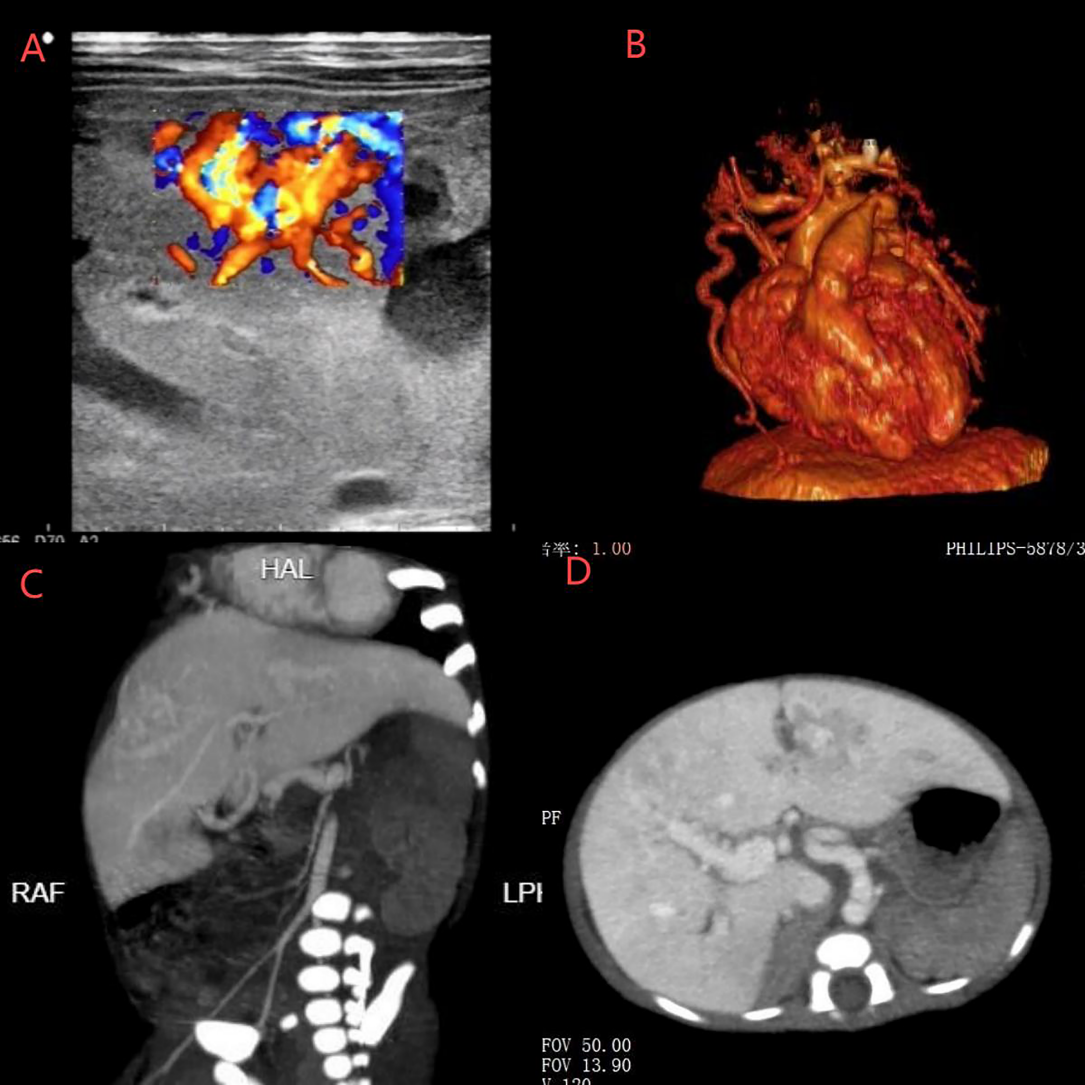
(A) Ultrasound showed cavernous transformation of the portal vein. (B) CT angiography revealed a dilated and tortuous right internal thoracic artery entering the liver. (C,D) CT identified hepatic artery-right portal vein fistula, hepatic portal-hepatic vein (left, middle, right) fistula, and right internal thoracic artery-left portal vein fistula.
